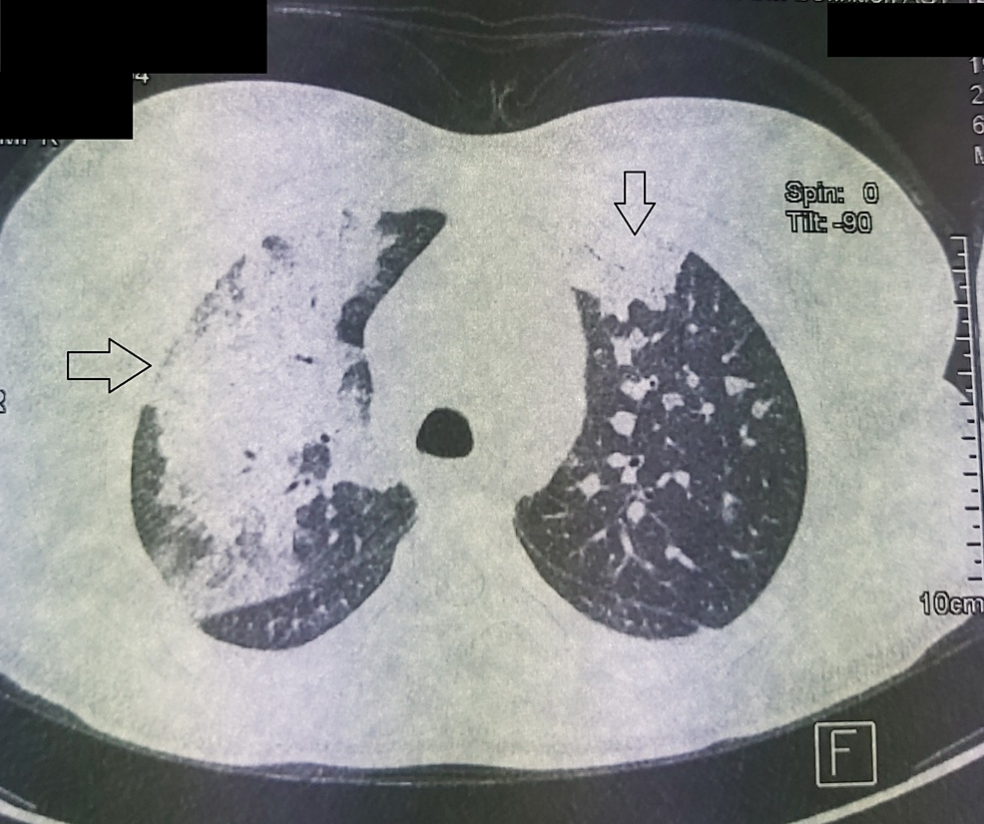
Chest CT axial section, showing bilateral alveolar-interstitial involvement
